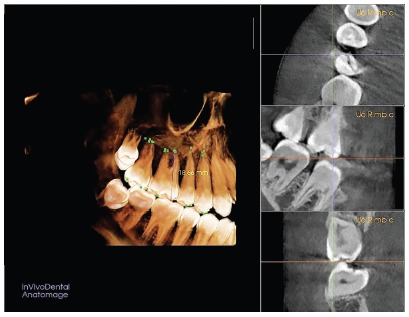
The three-dimensional determination of mesio-buccal cusp landmark of permanent maxillary right first molar (U6 R mbc) on the CBCT volumetric image.
